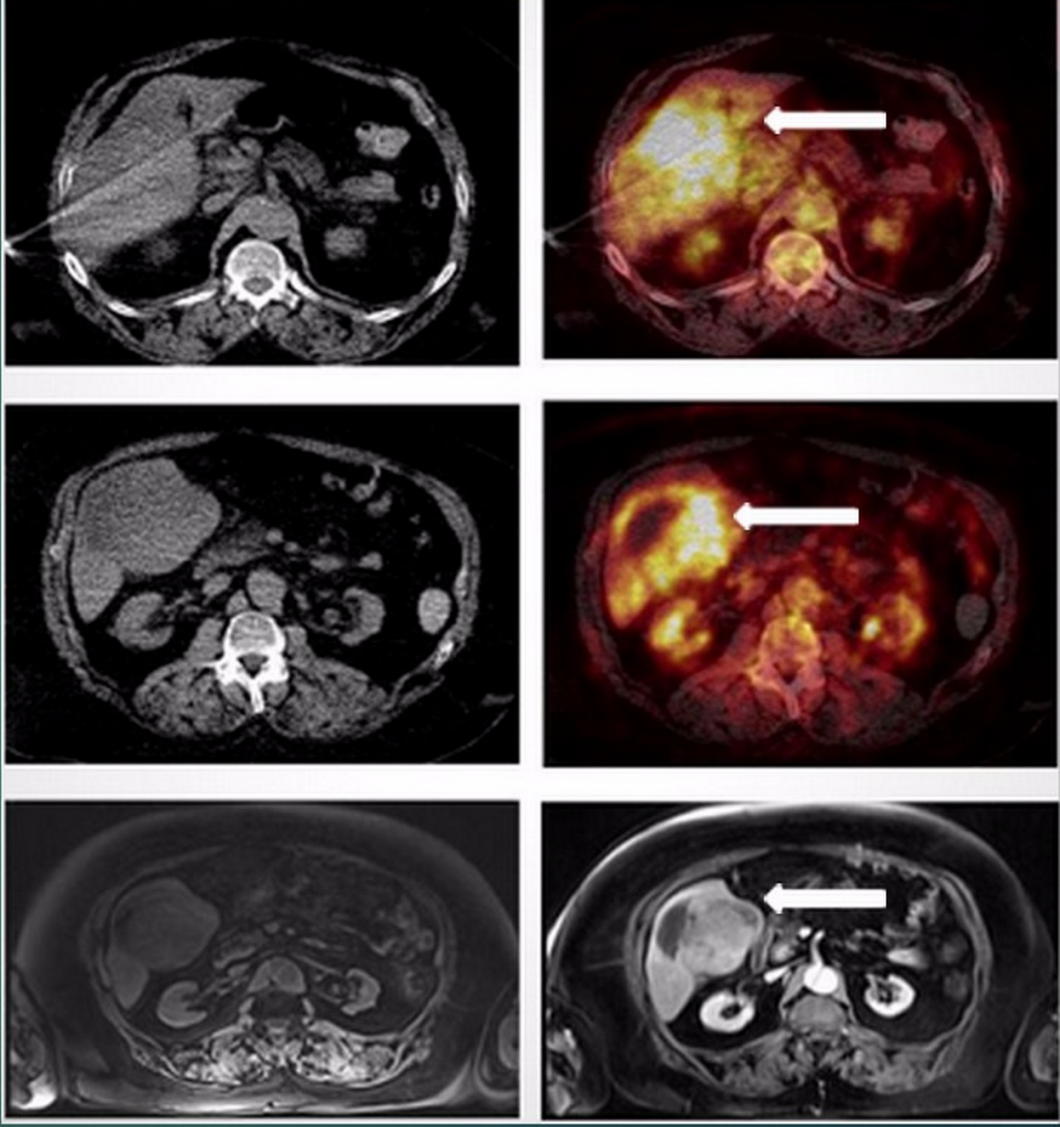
FDG-PET/CT and abdominal MRI The PET-CT study demonstrates an infiltrative right inferior hepatic lobe lesion with increased FDG activity (see arrow). There is increased T1 signal with heterogeneous increased T2 signal and heterogeneous post-contrast enhancement on axial T1-weighted VIBE images (see arrow).​
